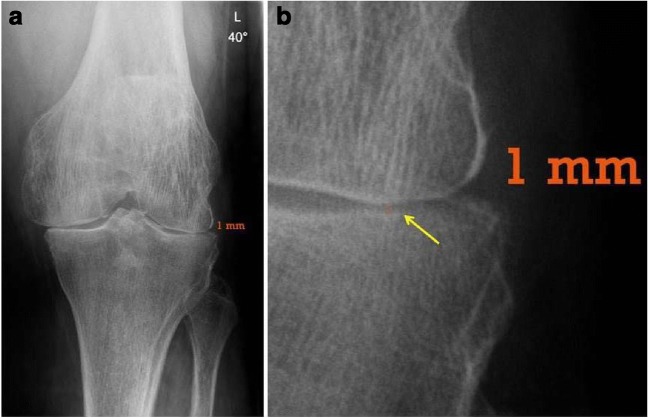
Example of weight-bearing flexed radiographs (Schuss-view) and of the measurement of the most pronounced narrowing of the joint space width; a overview; b detail
